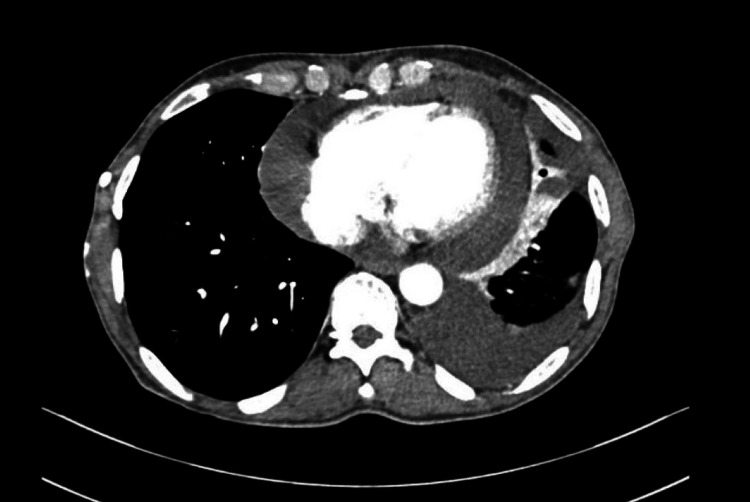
Contrast-enhanced CT thorax showing large pericardial effusion with a left-sided pleural effusion.
